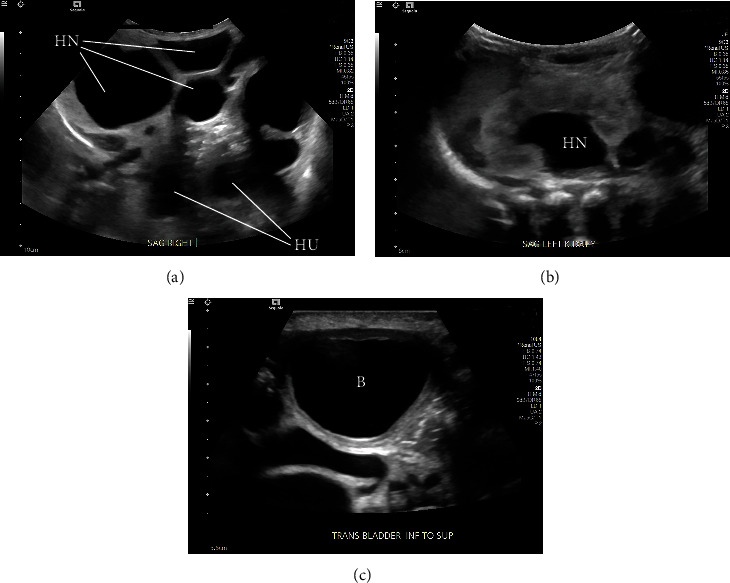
Renal and bladder sonography showed a severe (a) right hydroureteronephrosis, (b) moderate left hydroureteronephrosis, and (c) thick wall urinary bladder. HN: hydronephrosis; HU: hydroureter; B: urinary bladder.
